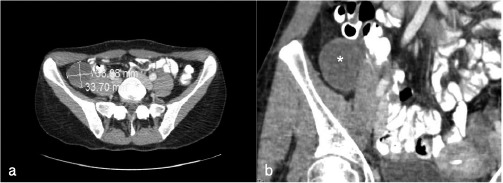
Figure 1a-1b: A 38-year-old woman with right fossa syndrome. a. Coronal computed tomography (CT) image of the abdomen showing a cystic formation in the right flank (33,70 x 38,08 mm). b. Frontal CT image sowing a cyst of 40 Hounsfield Units (asterisk).
